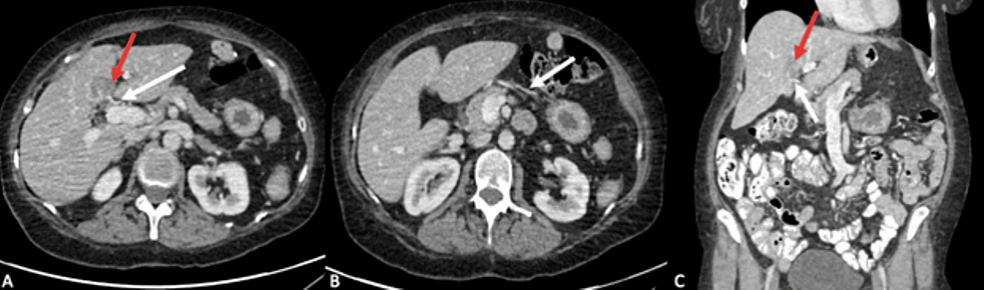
Contrast-enhanced abdominal CT Portal phase contrast-enhanced abdominal CT images (A, B, C) show an aberrant course of the right gastric vein (white arrows) draining into the hepatic segment IVb, consistent with an ARGV. A focal hypodense perfusion defect (red arrows) is visible in this location, which is traversed by blood vessels originating from the right gastric vein. ARGV: aberrant right gastric vein
